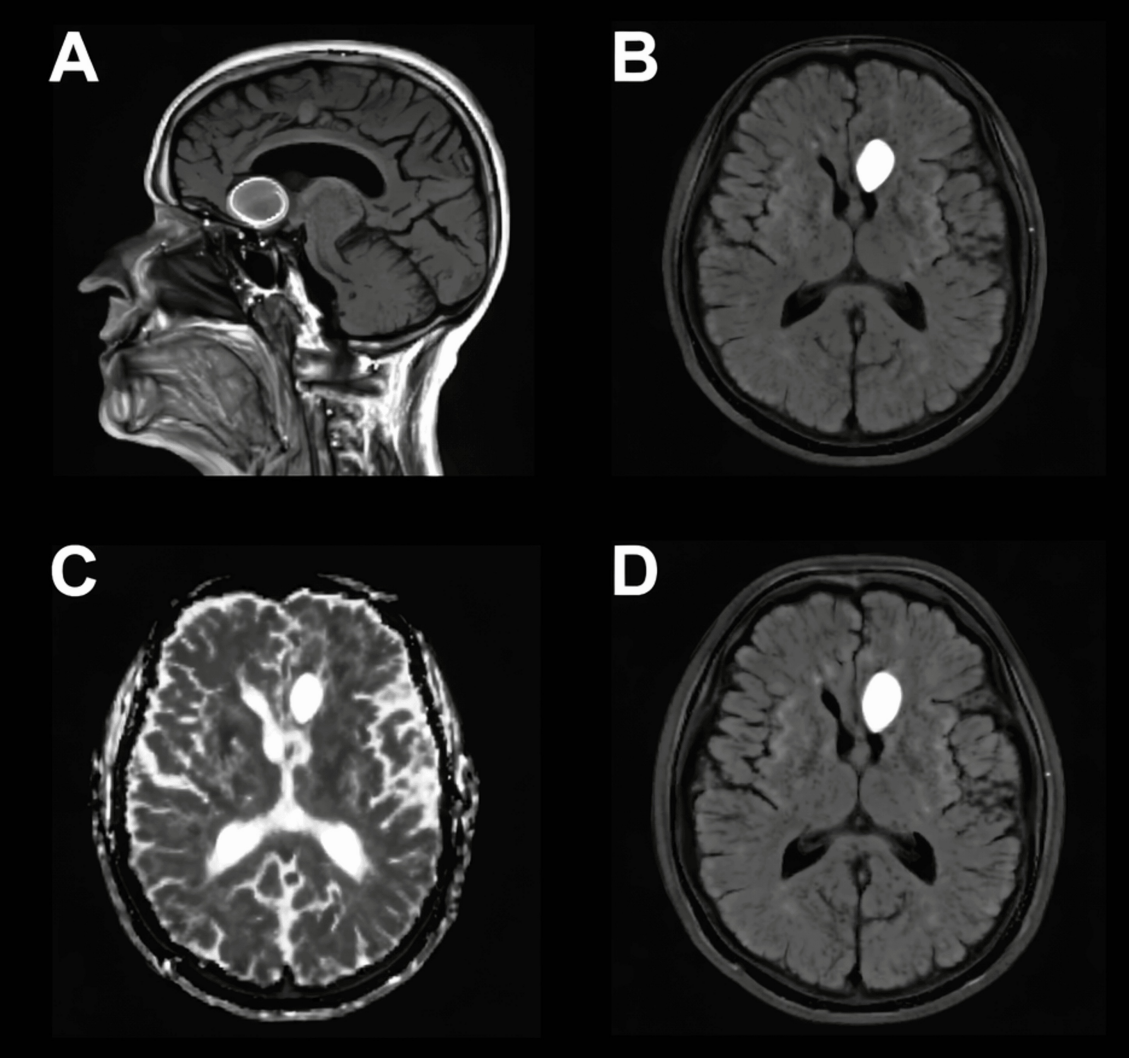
Follow-up brain MRI one month after initial presentation. A. Sagittal T1-weighted MRI demonstrating the intraparenchymal lesion in the left inferomedial frontal lobe. B. Axial FLAIR sequence showing the residual hyperintense cystic component within the lesion. C. Axial T2-weighted MRI highlighting internal cystic signal characteristics. D. Axial FLAIR MRI depicting interval reduction in lesion size with resolution of perilesional edema. FLAIR, fluid-attenuated inversion recovery
